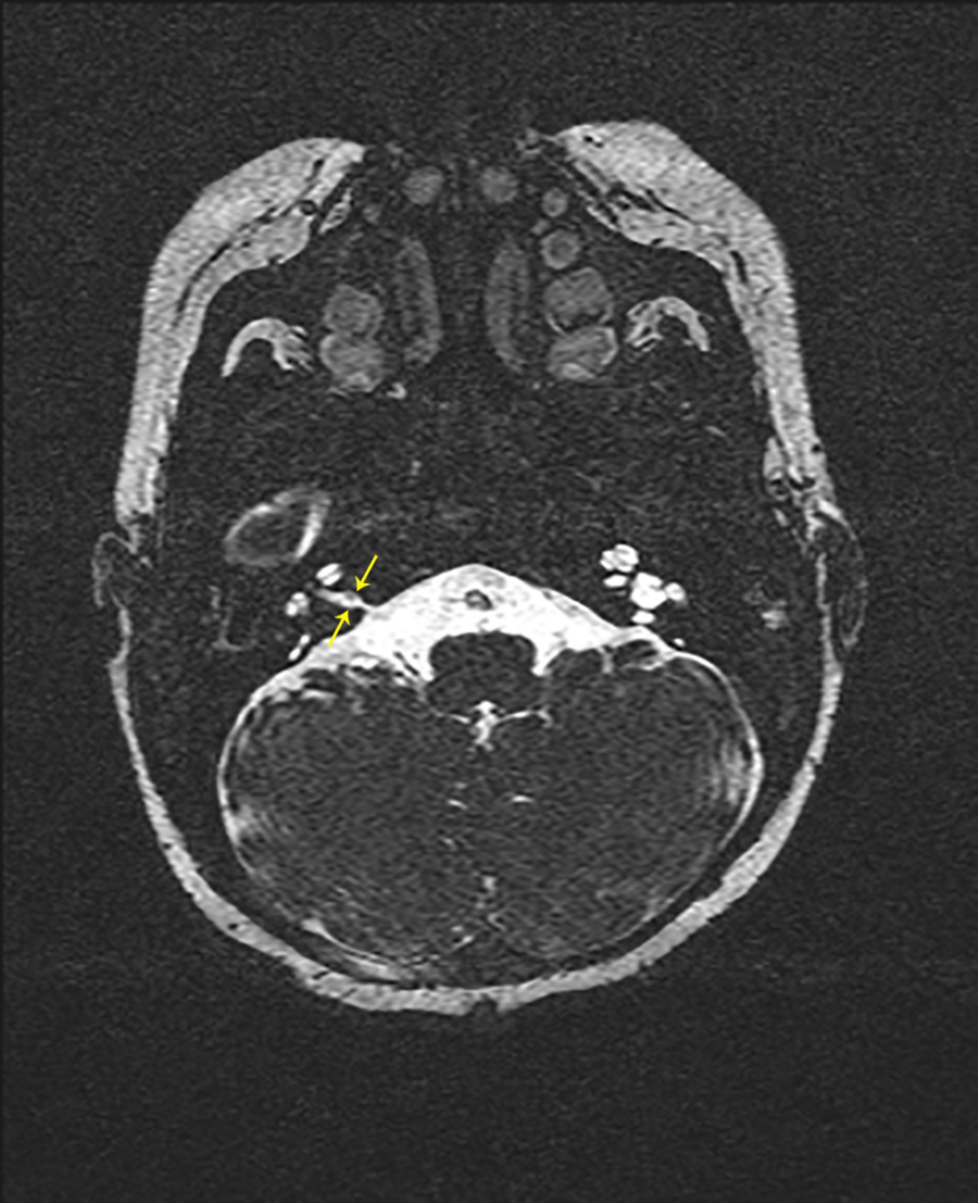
MRI of the internal auditory canals without IV contrast. CISS sequence, axial cut revealing stenotic right IAC (yellow arrows) without visible CN VIII. MRI - magnetic resonance imaging
